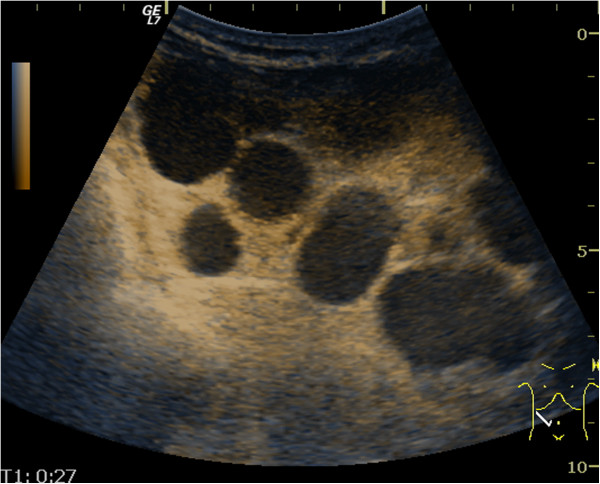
Contrast-enhanced ultrasound - arterial phase (27 seconds) – cystic mesenteric masses - arterial rim enhancement.
